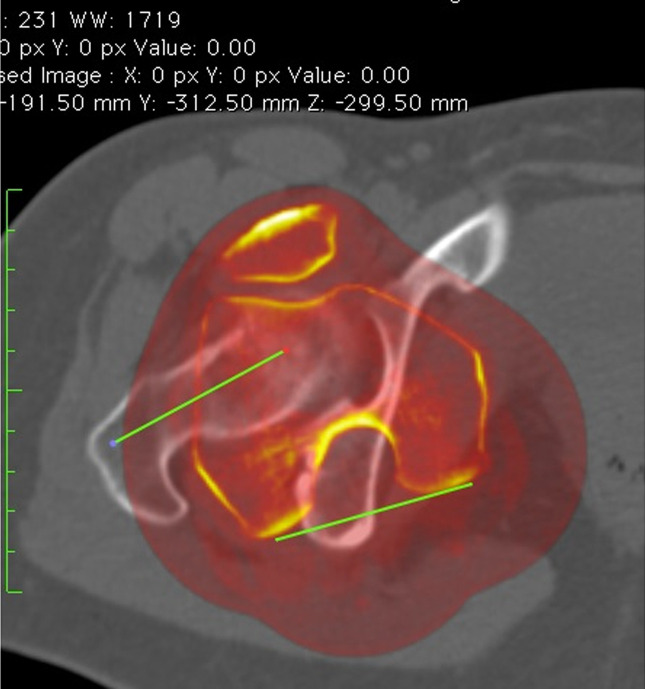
Femoral torsion. Superposition of two CT slices. The first slice is in the proximal femur, and the second slice is in the distal femur. Femoral torsion is measured as the angle between a line in the femoral neck axis and a line joining the posterior borders of both condyles.
